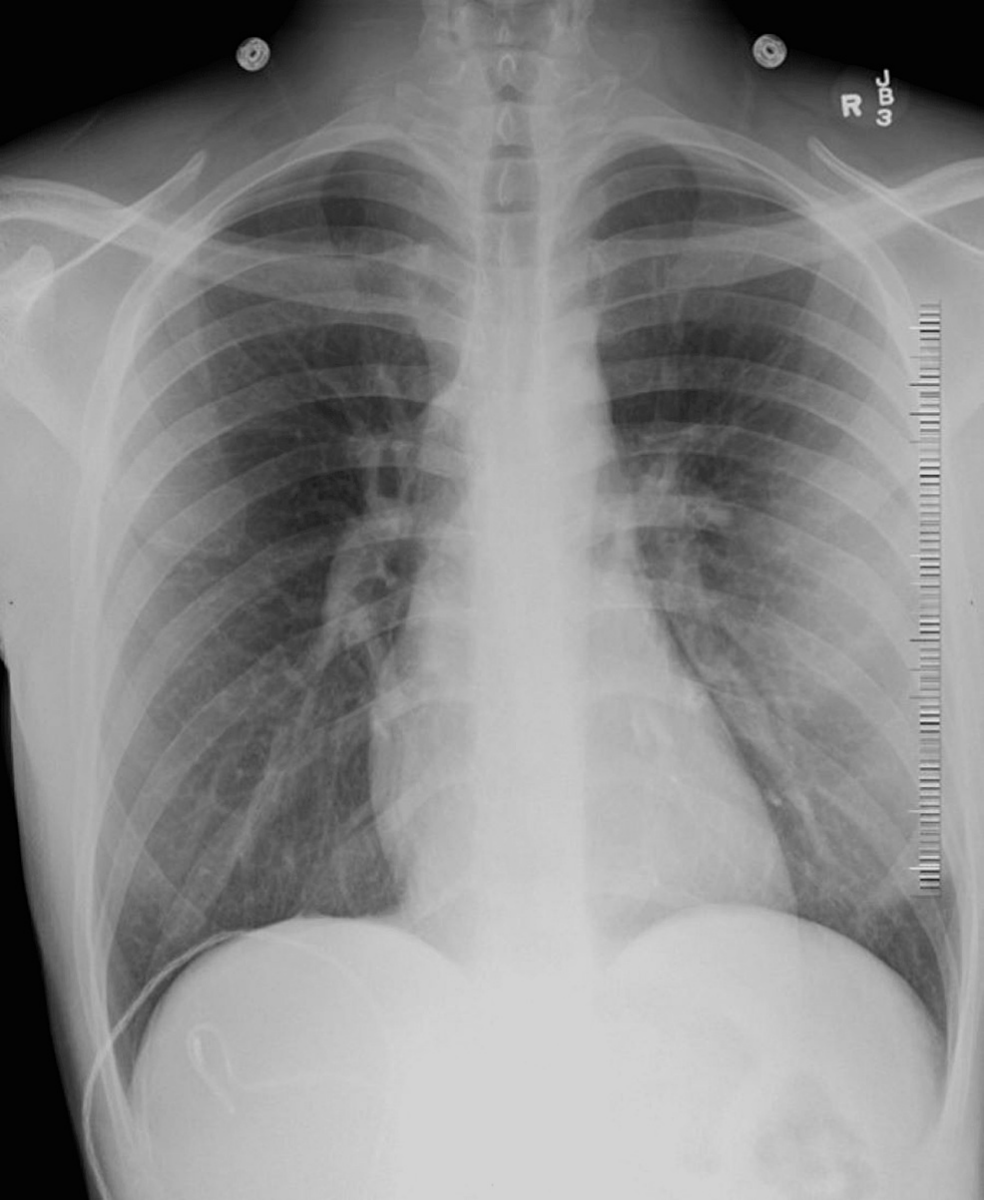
No acute abnormality on the chest X-ray
